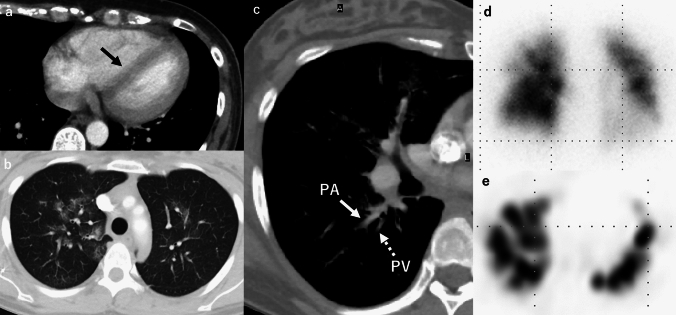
Amniotic fluid embolism (AFE). A woman in her 30 s developed continued bleeding following labor induction and fetal delivery which was controlled by uterine artery embolization (UAE). Persistent hypoxemia was noted after the completion of the UAE. Contrast-enhanced CT revealed right ventricular and pulmonary artery dilation, along with leftward deviation of the interventricular septum (a). The lung fields demonstrated multiple GGOs predominantly in the perihilar regions (b). The peripheral pulmonary veins (PVs) appeared narrower than the corresponding pulmonary arteries (PAs), suggesting the presence of emboli at the level of the pulmonary capillaries (c). 99mTc-MAA lung perfusion scintigraphy revealed multiple fine band-like and wedge-shaped perfusion defects in both lungs, consistent with a peripheral pulmonary arterial embolism (d, e). Elevated zinc coproporphyrin levels and decreased C1 inhibitor activity were observed, leading to a diagnosis of AFE
